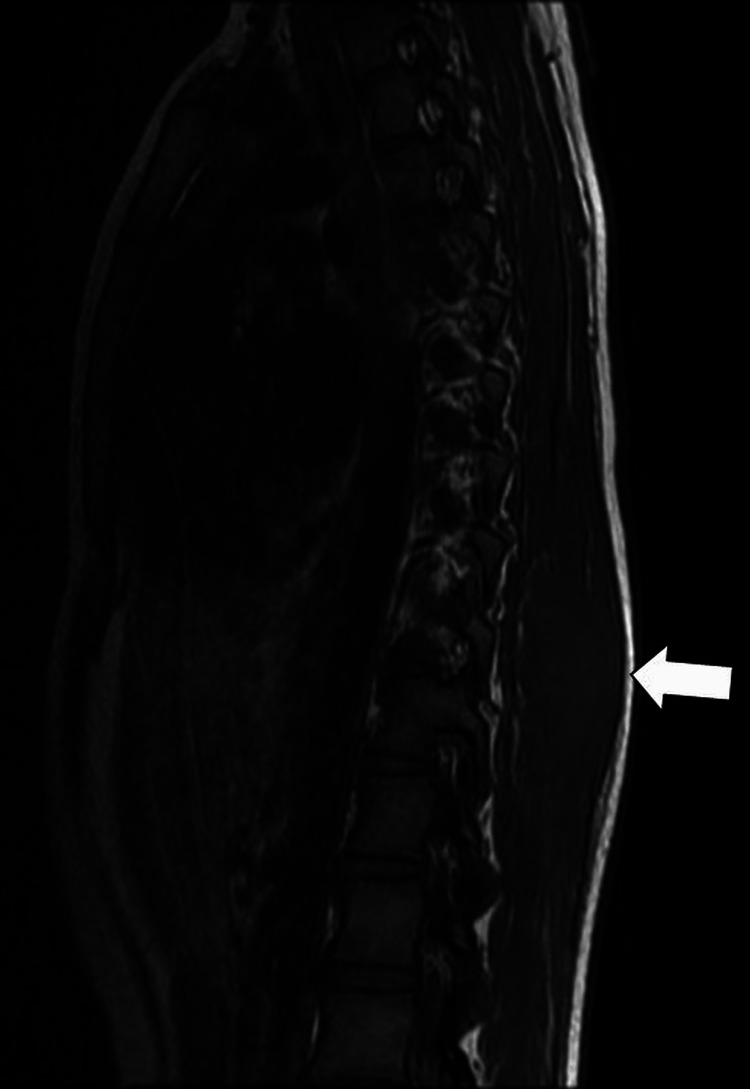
Saggital T1-weighted MRI shows a lesion (arrow) that has low to intermediate signal intensity and isointense to muscle when it extends from the D9 to the D12 vertebrae.
